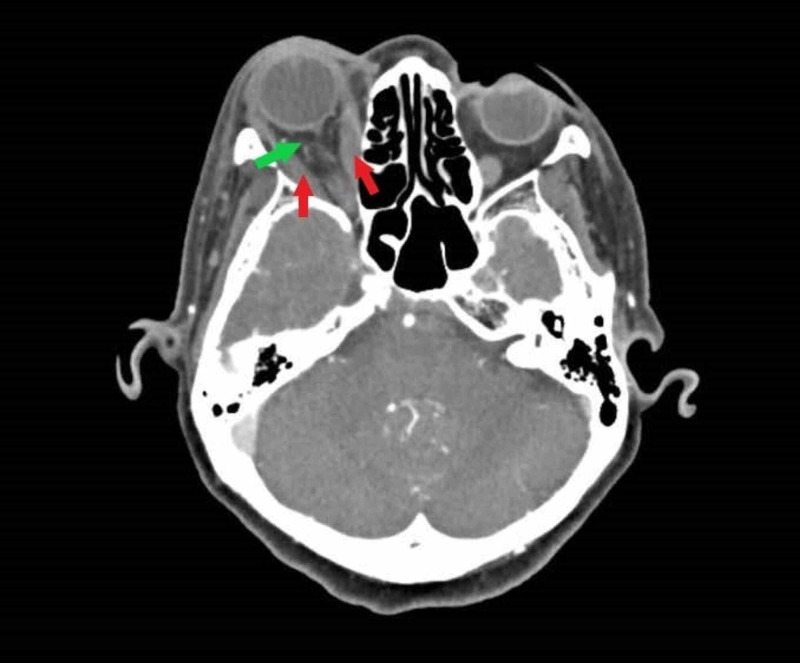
Axial computed tomography of the brain and orbit showing thickened extraocular muscles (red arrow) and streakiness of intraconal fat (green arrow), with normal concavity of the superior ophthalmic vein and cavernous sinus.
